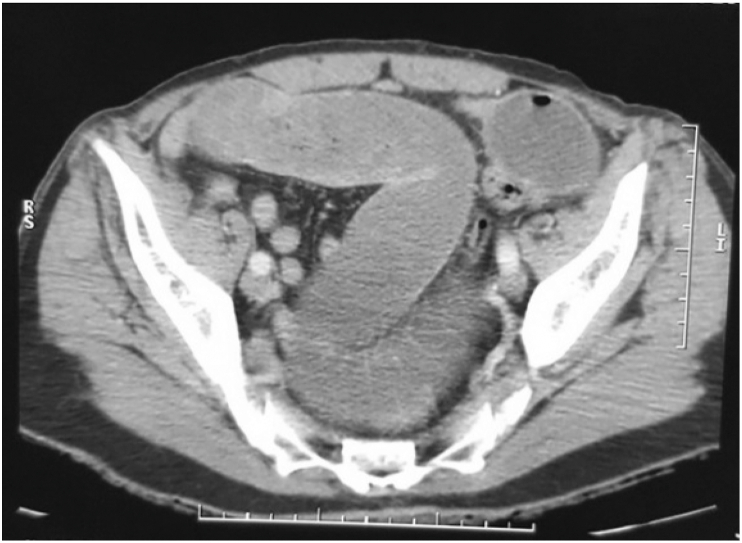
CT scan section showing the retrocecal location of the distal ileum.
